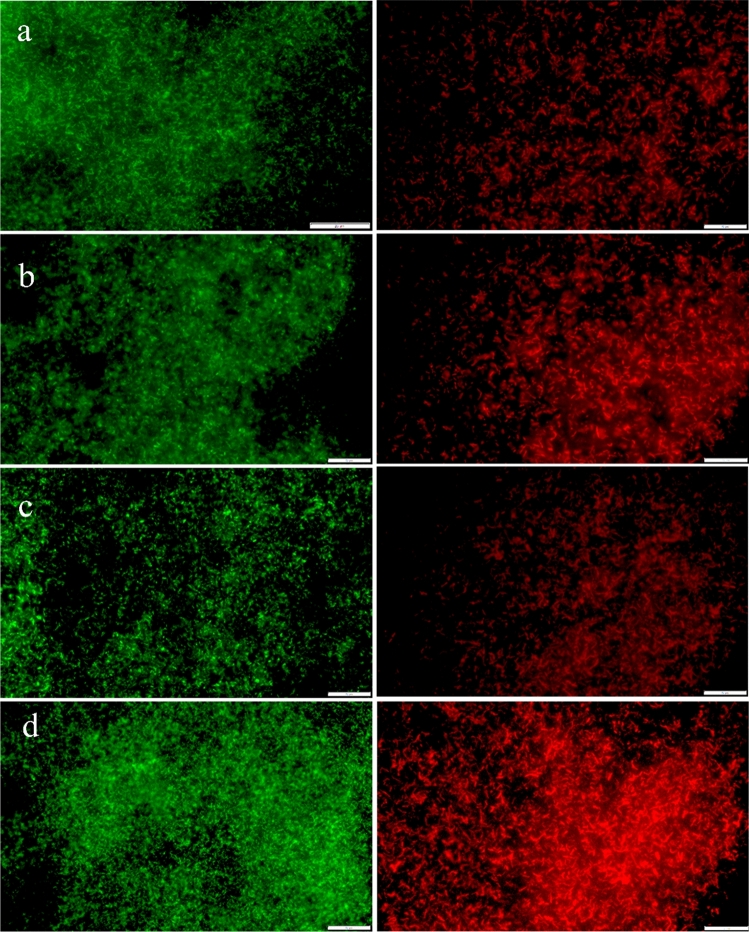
E. coli* bacteria marked with SYTO™ 9 (green) and propidium iodide (red) fluorescent dyes: a Ag@CT 0.54 µg/mL, b Ag@CT 6.47 µg/mL, c Ag@CB 0.54 µg/mL, d Ag@CB 6.47 µg/mL. Scale: 20 µm
